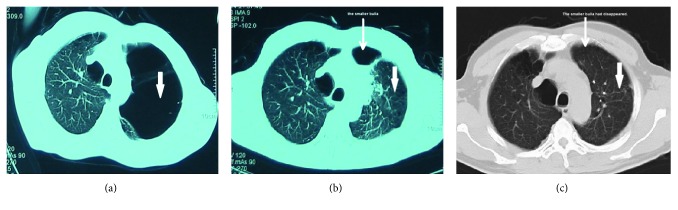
Case 3 (patient 2) of GPB treated by IBAP as shown by CT scan. The 52-year-old male patient with bilateral pulmonary bullae and left GPB (thick arrow) (a). Seven days after IBAP in July 2006, the GPB closed completely (thick arrow), while a smaller bulla in the same side appeared (thin arrow), which may be previously compressed by the target GPB (b). Six years after IBAP, the left GPB did not recur (thick arrow) and the smaller one disappeared (thin arrow) (c).
